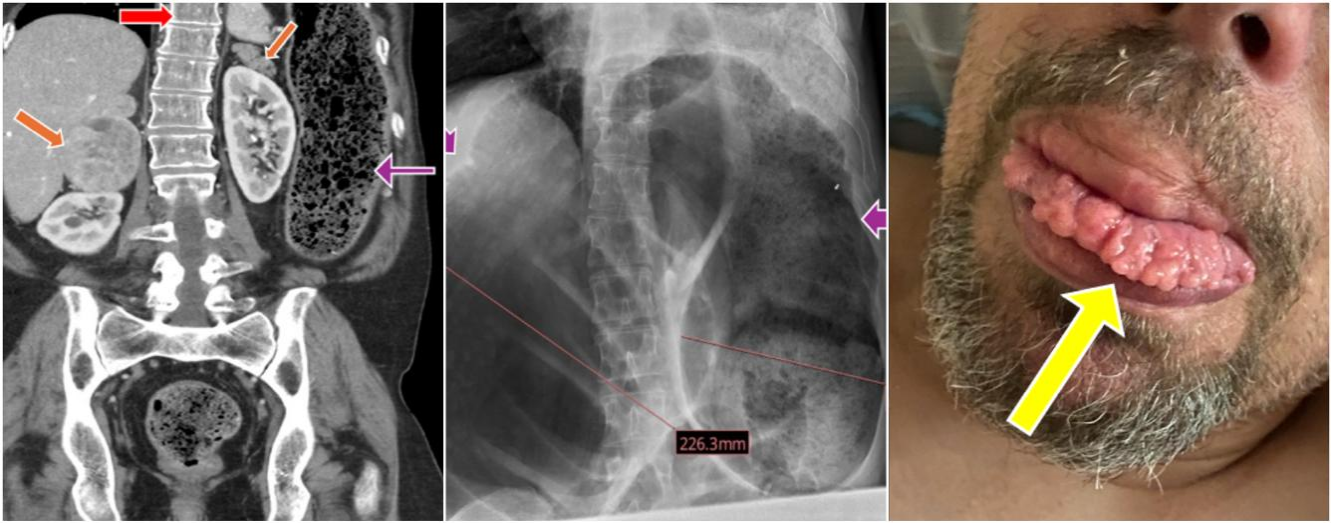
CT scan of the abdomen shows bilateral adrenal masses, lateral scoliosis and dilated colon on abdomen X-ray & mucosal neuromas on the tongue.
